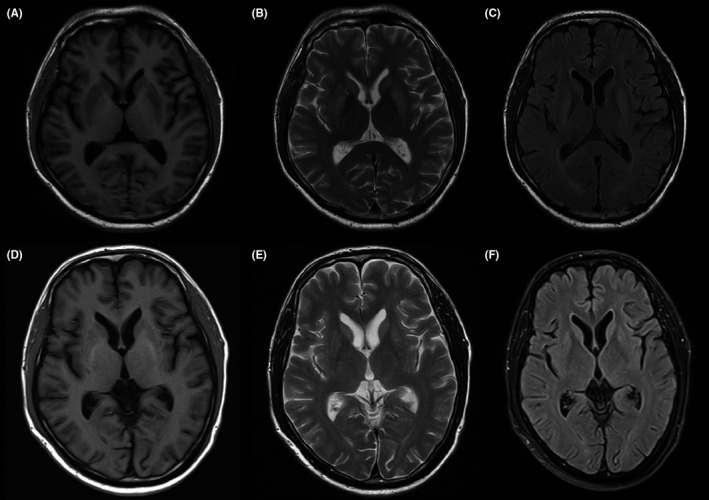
Axial MRI images at admission show lesions in bilateral caudate head and putamen: (A) hypointensity on T1‐weighted images, (B) hyperintensity on T2‐weighted images, (C) hyperintensity on T2‐weighted fluid‐attenuated inversion recovery sequences. Repeated MRI images 3 mo later show lesions with obvious improvement in bilateral caudate head and putamen: (D) hypointensity on T1‐weighted images, (E) hyperintensity on T2‐weighted images, (F) hyperintensity on T2‐weighted fluid‐attenuated inversion recovery sequences
